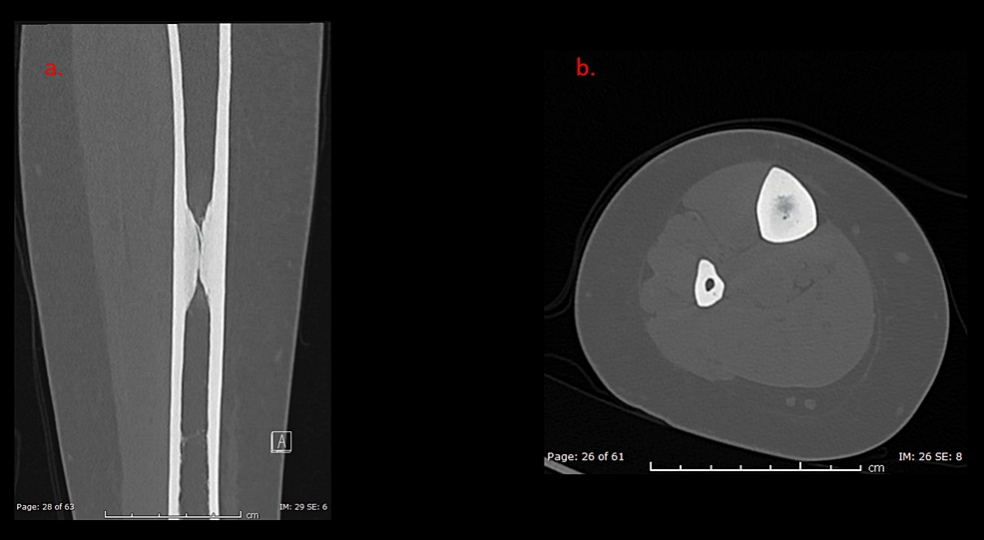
Coronal (a) and axial (b) computed tomography examination of the right leg in bone window. Focal circumferential endosteal/medullary solid cortical thickening of the mid-tibial shaft. No evidence of an old fracture line, cortical nidus or sequestrum, periosteal reaction, or soft tissue component.
